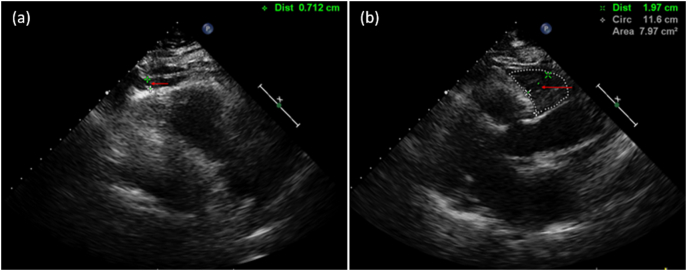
Parasternal long-axis section of the left ventricle showing epicardial adipose tissue in the apex and bottom of the heart (arrow points). (a). EAT thickness near the apex. (b) EAT thickness near the heart base. EAT: epicardial adipose tissue.
